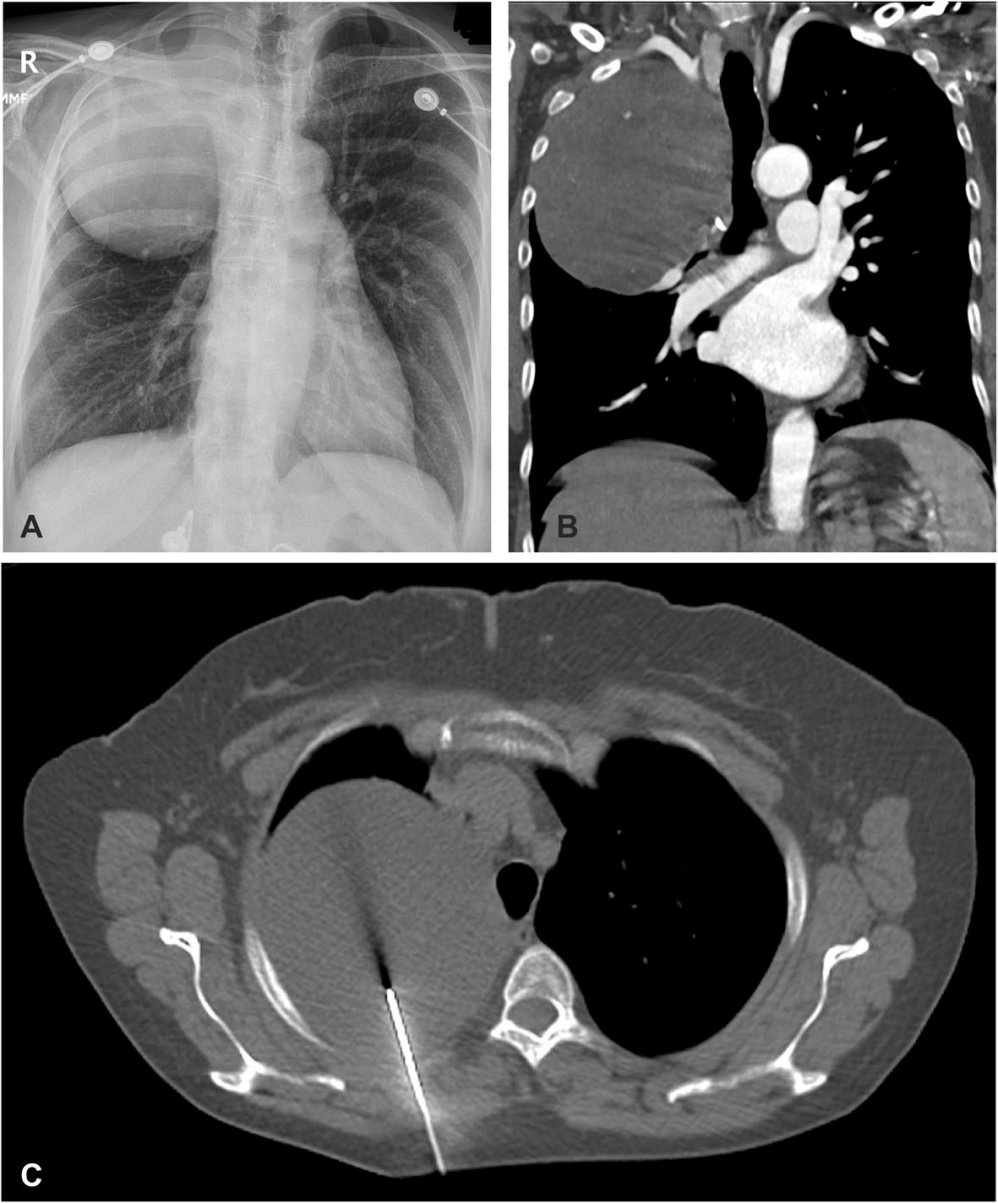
Radiology images of the pulmonary mass. Chest X-ray (A) and CT (B and C) demonstrate a well-circumscribed right upper lung mass that abuts the trachea but does not compress the airway. The lesion shows broad pleural abutment without invasion of the chest wall or ribs. The tiny hyperdense focus in the central upper portion of the mass represents calcium deposits (B)
